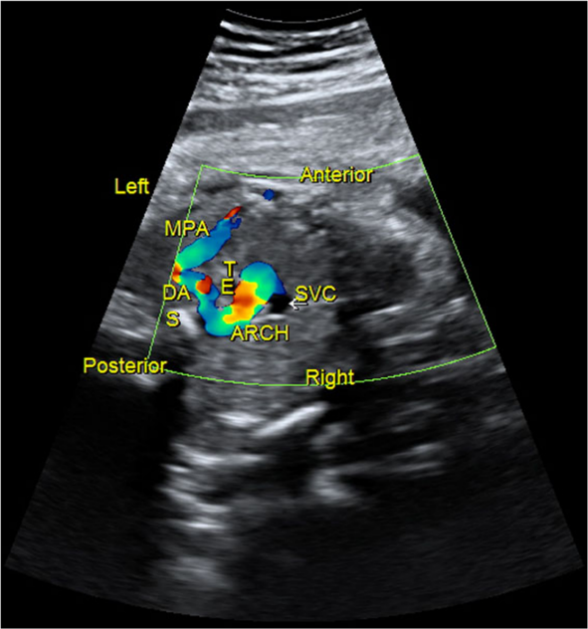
Sonographic images of a 23-gestational-week fetus diagnosed with right aortic arch. In the 3VT, the confluence of the DA and aortic arch formed a “U”-like structure, surrounding the trachea and esophagus. This was a characteristic sign of right aortic arch. 3VT, three-vessel-trachea view; DA, ductus arteriosus; E, esophagus; MPA, main pulmonary artery; S, spine; SVC, superior vena cava; T, trachea
